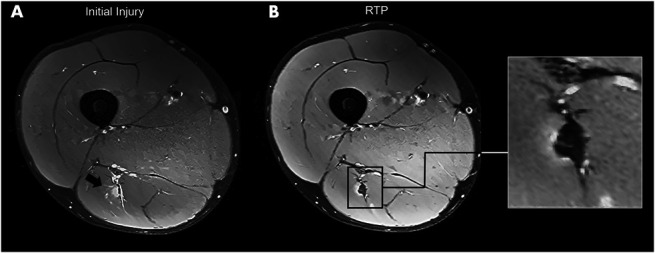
MRI at initial injury and at RTP of biceps femoris long head (BFlh) from a similar participant (A) Axial proton density (PD) Dixon MRI of the initial injury showing presence of edema (arrow) (B) Axial PD Dixon MRI at RTP showing the progression of fibrosis (arrow) with presence of edema.
